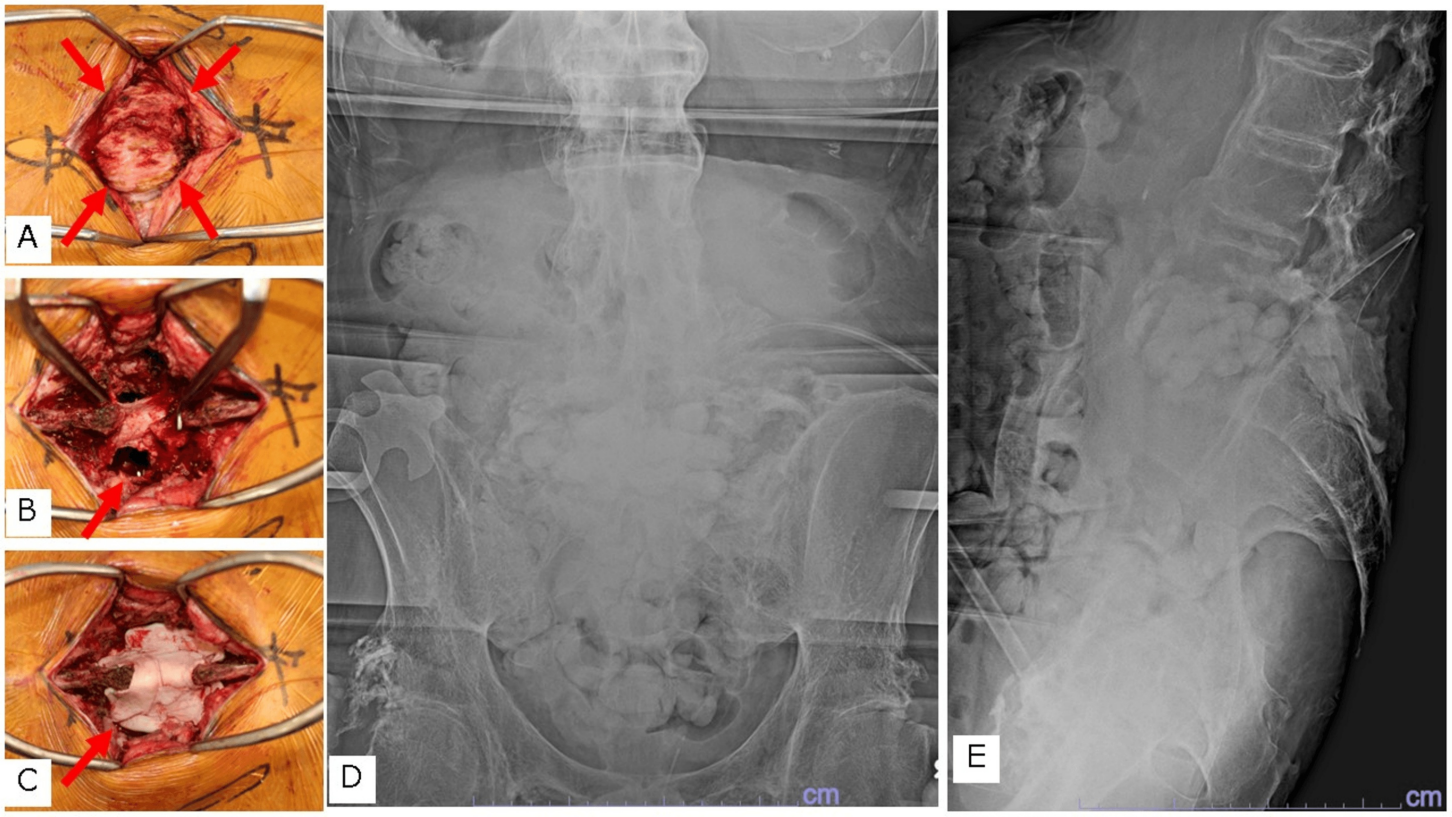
Intraoperative image and postoperative X-ray of the first surgery A. Enbloc resection of the encapsulated inflammatory soft tissues was performed as much as possible. B. Debridement of the posterior soft tissue and intervertebral space was performed. C. A cement spacer was placed in the intervertebral and interlaminar space to provide stability. D. Anteroposterior postoperative X-ray; E. Lateral postoperative X-ray
